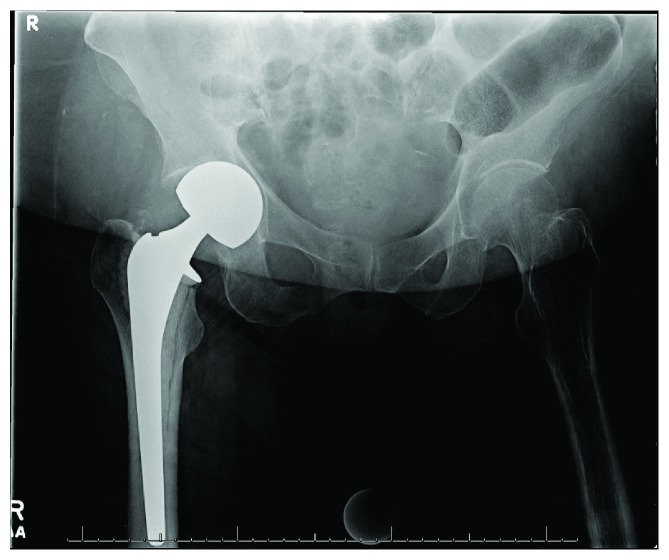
AP pelvis radiograph obtained 2 weeks postop demonstrating joint space narrowing and medialization of femoral component.
